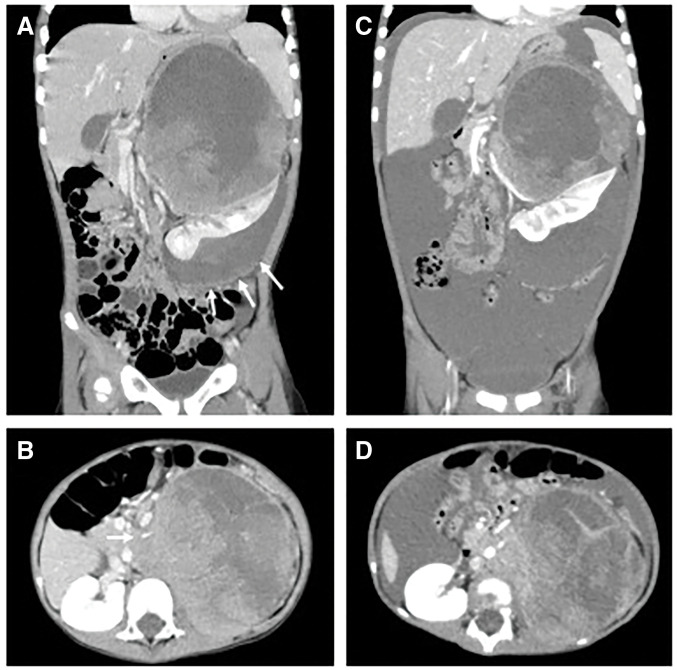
Contrast-enhanced abdominal CT findings. (A, B) Initial contrast-enhanced abdominal CT revealed a well-defined 17 × 11 × 9.5-cm heterogeneous mass compressing the left kidney. A low-density area lateral to the kidney encapsulated by a membrane suggestive of a hematoma was also identified (white arrows in A). The left renal artery and vein were encased between the tumor and enlarged para-aortic lymph nodes, consistent with IDRFs (white arrow in B). (C, D) Contrast-enhanced CT performed 7 days after the initiation of induction chemotherapy revealed increased ascites and findings suggestive of tumor rupture. IDRF, imaging-defined risk factor
